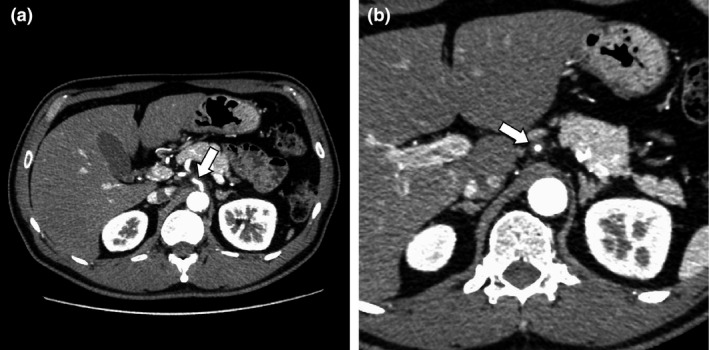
Follow‐up abdominal contrast‐enhanced CT 6 days later. The dilatation of true lumen of left gastric artery was shown in the follow‐up CT (a, b, arrows).
